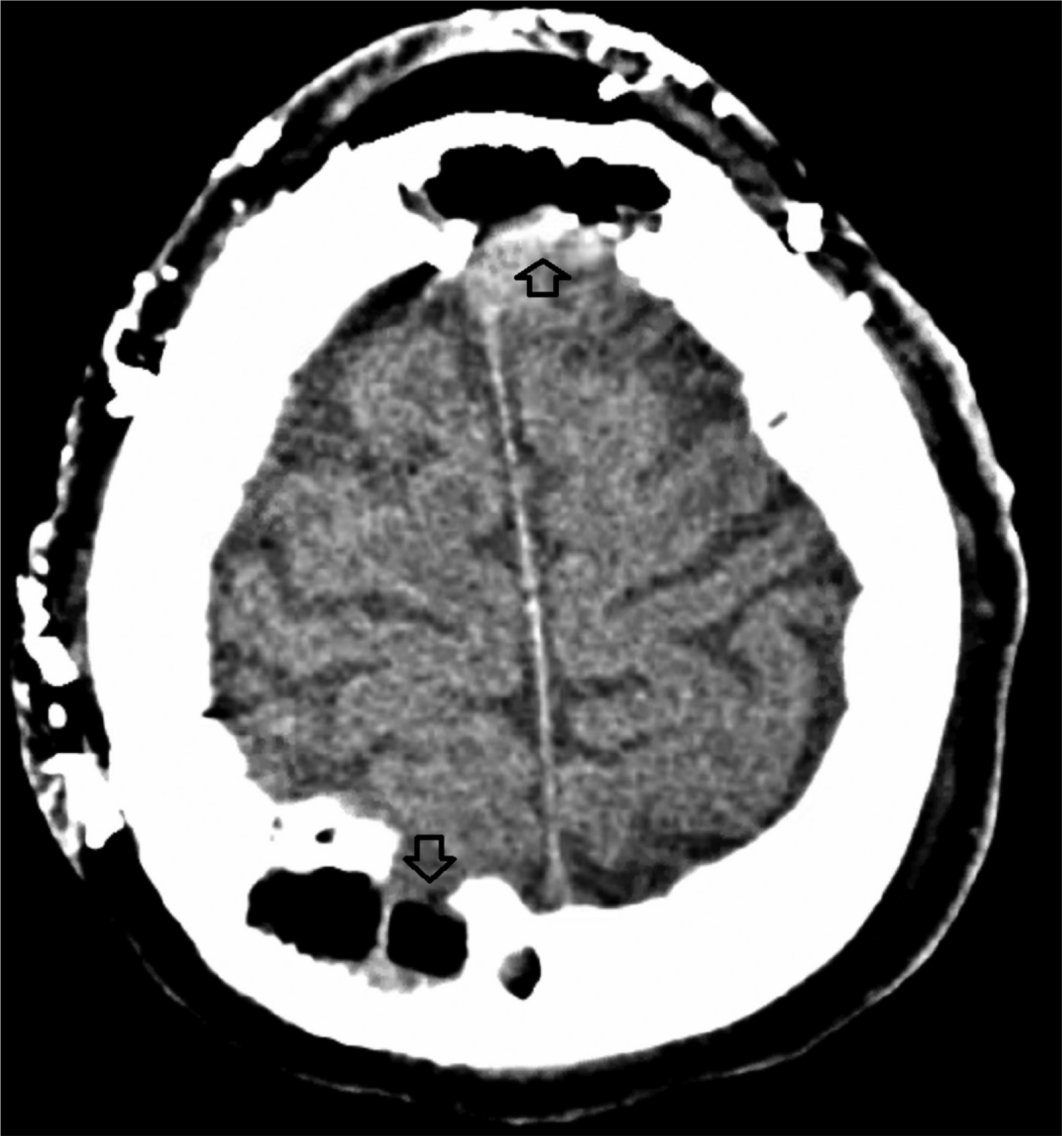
Head CT scan without contrast axial view at 3‐month postoperative follow‐up visit revealing a gross total resection of all calvarial lesions.
